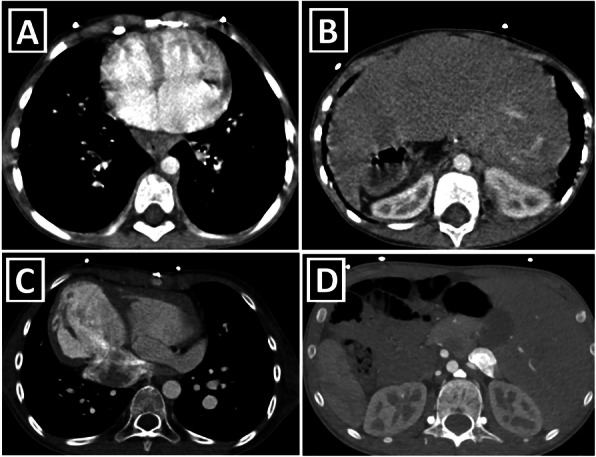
PLSVC with heterotaxy-1 (situs ambiguus and situs inversus). a, b Axial CT images depict mesocardia (a), midline liver (b), right-sided stomach (b), and absence of spleen (b) in a patient with situs ambiguus and PLSVC (not shown). c, d Axial CT images show situs inversus characterized by dextrocardia (c), right-sided stomach and spleen (d), and left-sided liver (d) in a patient with double SVC (not shown)
